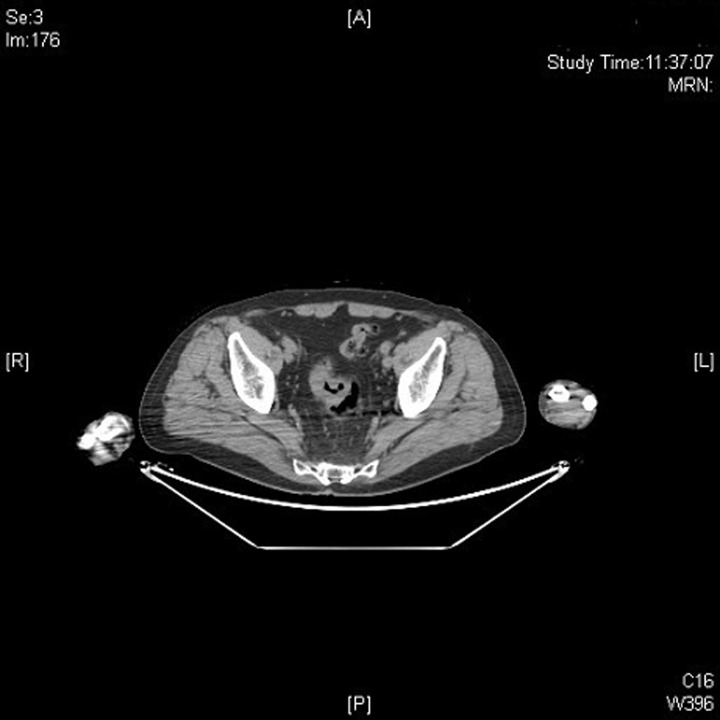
computed tomography (CT) that identifies the primary lesion at the level of the rectum
